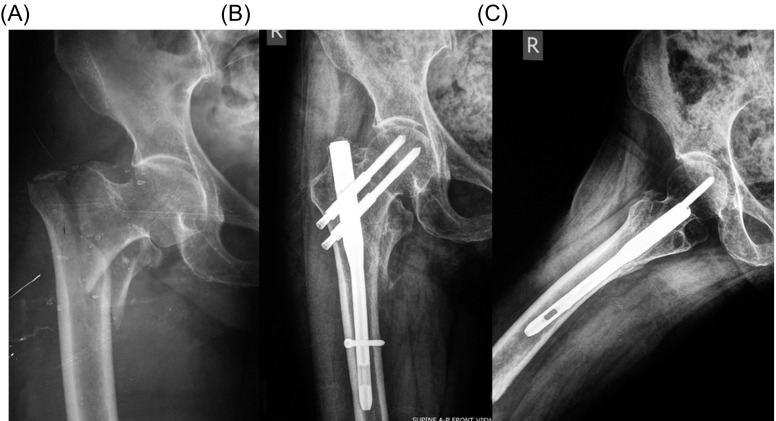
(A) Anteroposterior radiograph of the right hip of a 65-year-old woman shows an AO/OTA-31-A2 fracture. (B) Anteroposterior and (C) lateral radiographs of the right hip one year after surgical treatment show fracture healing. The patient was alive with excellent function.
